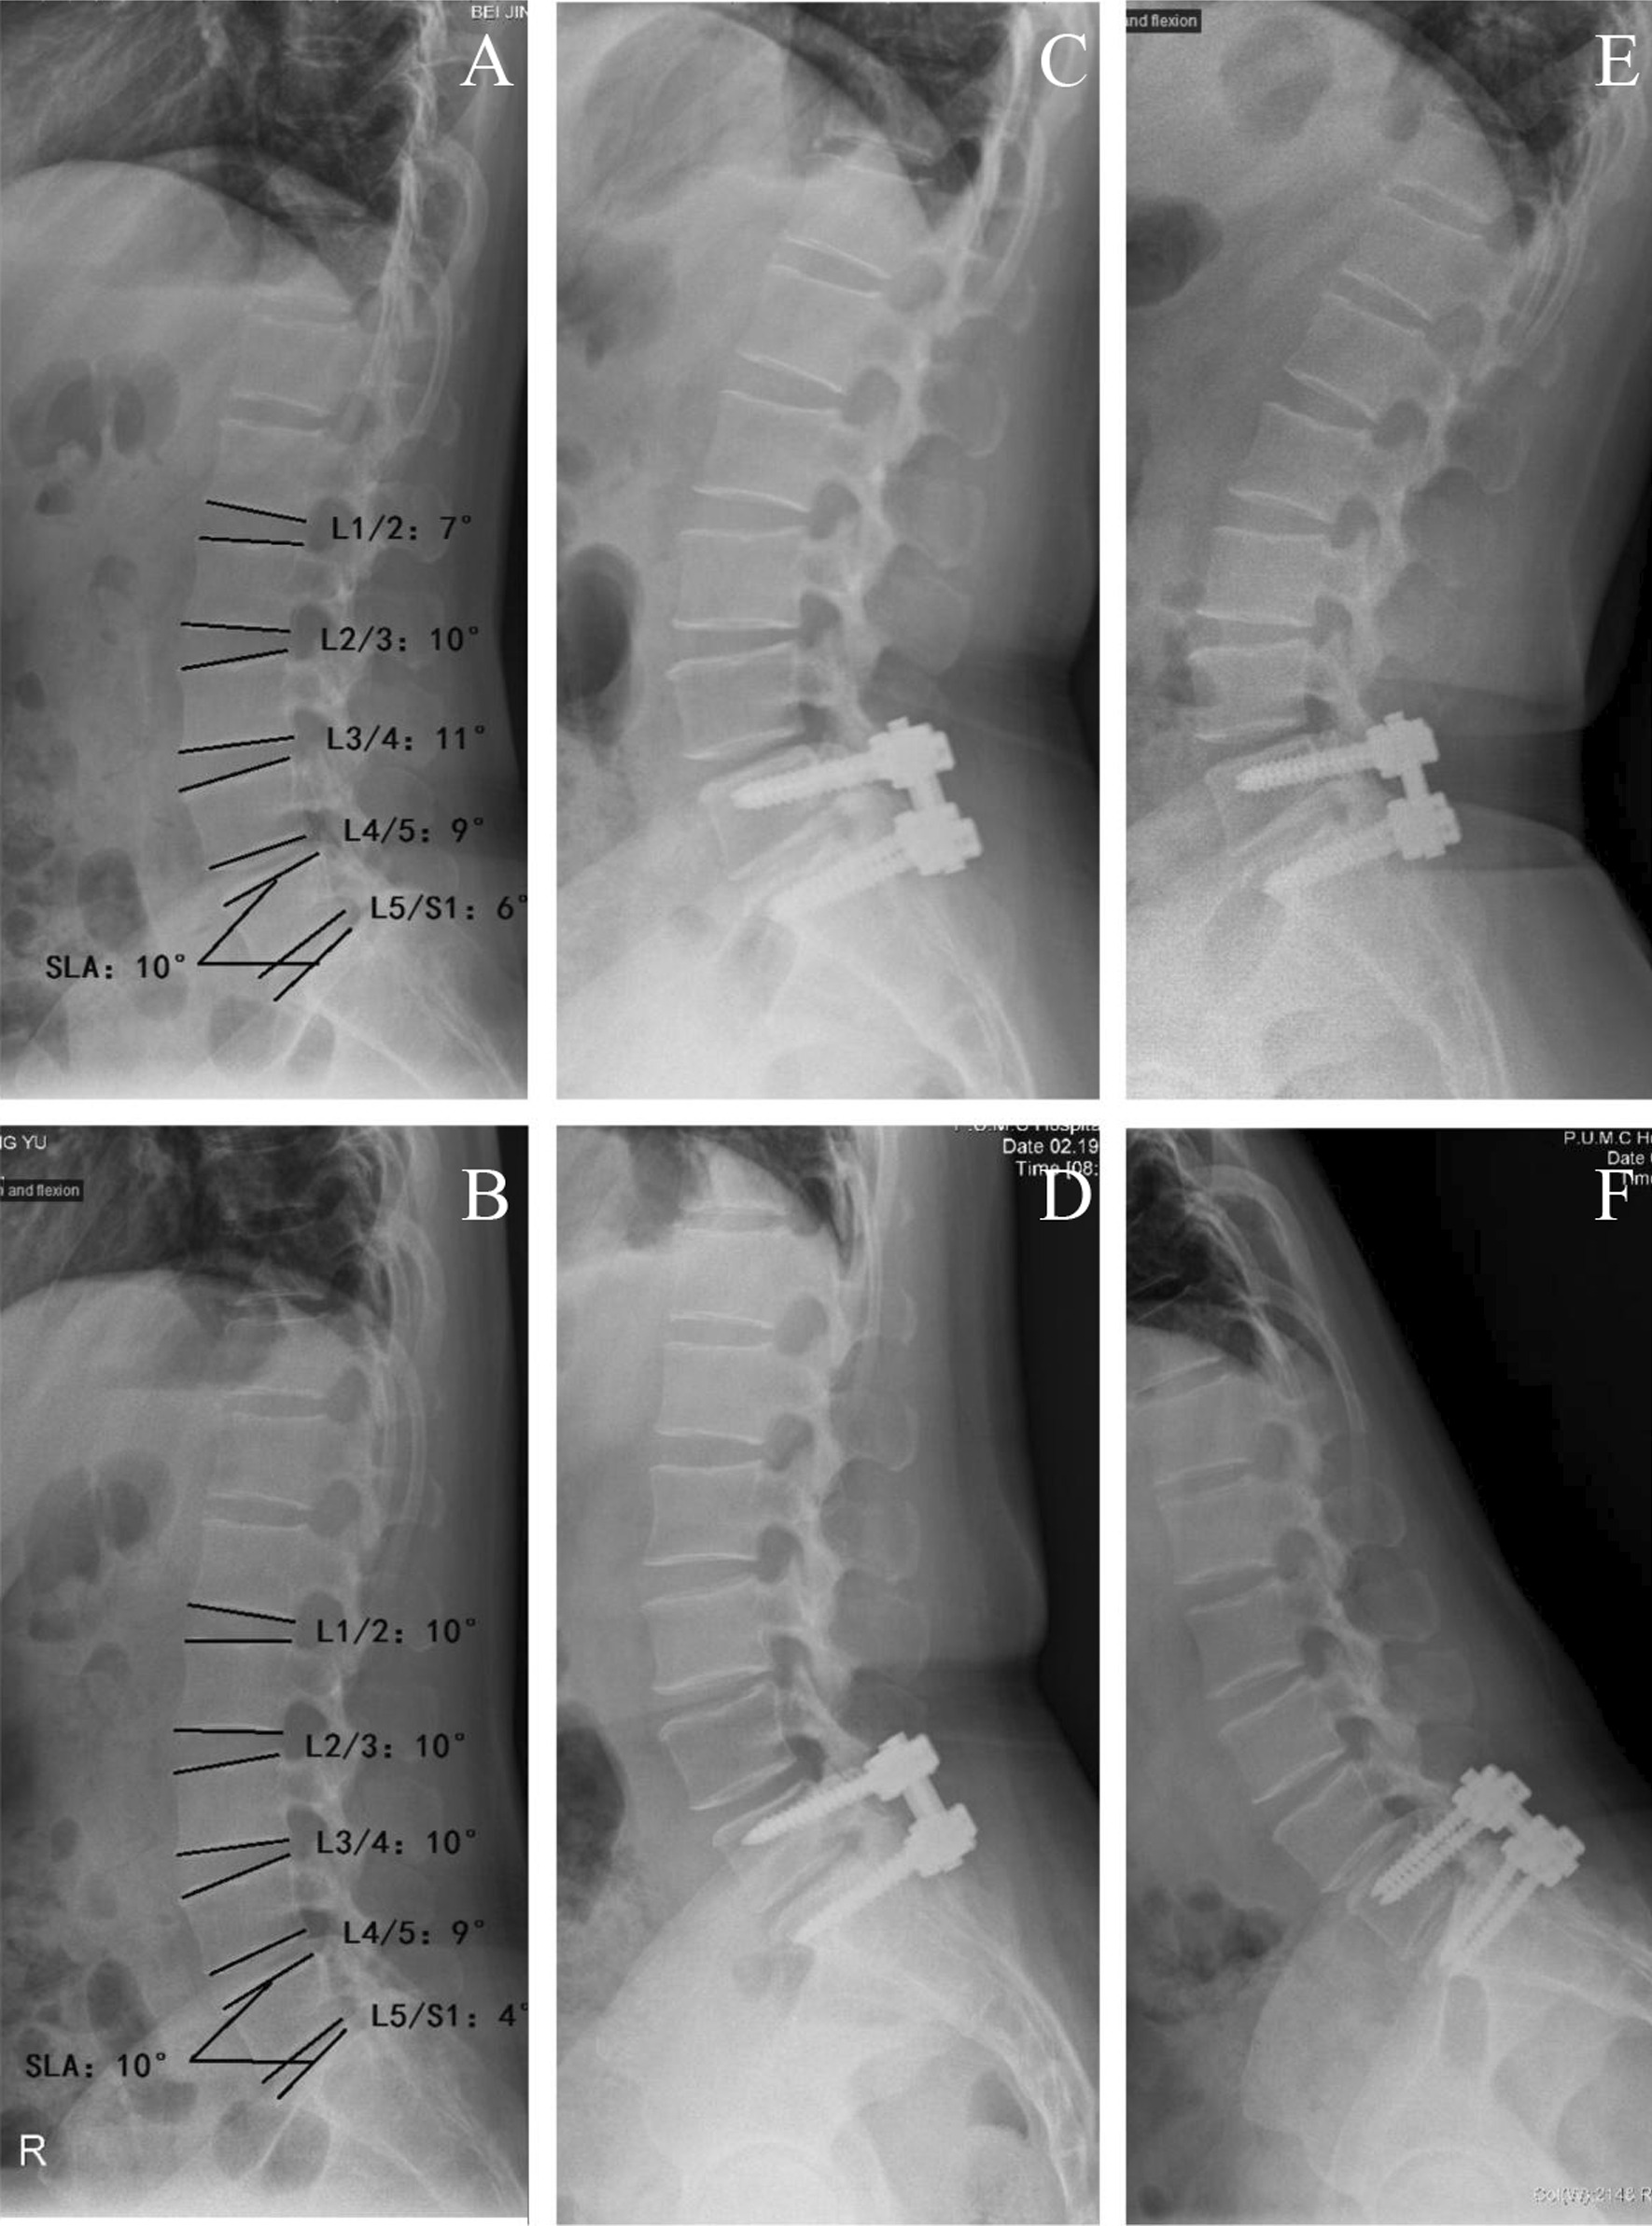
Preoperative, 6-month and 12-month lateral lumbar extension-flexion radiograph. A and B Preoperative lateral lumbar extension-flexion radiograph. Measurement of intervertebral angle and segmental lordosis angle are demonstrated in the picture. △intervertebral and ROM-SLA = extension-flexion. C and D 6-month lateral lumbar extension-flexion radiograph. E and F 12-month lateral lumbar extension-flexion radiograph
